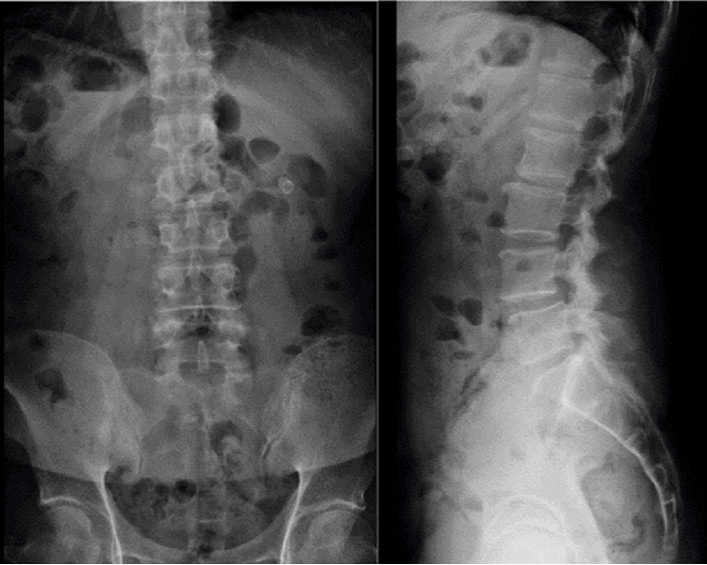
Preoperative X-ray film. These anteroposterior and lateral views reveal grade 1 spondylolisthesis of L4 over L5.
